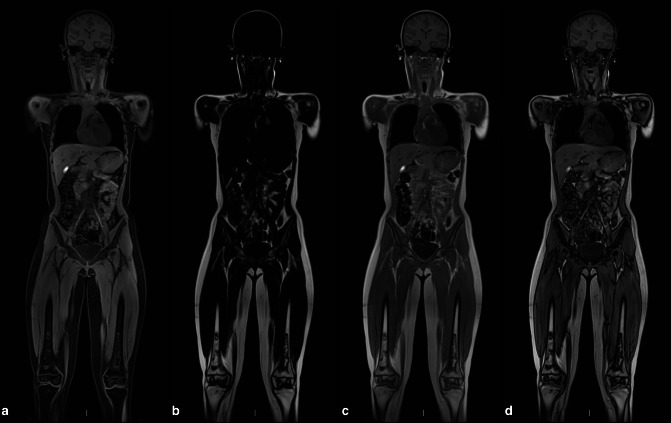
Whole-body coronal T1 Dixon MRI examinations showing water only (A), fat only (B), in-phase (C) and out-of-phase (D) sequences. Focal regions of medullary osteonecrosis can be seen in the distal femoral metaphases and epiphyses. The bone marrow otherwise returns normal signal with homogeneous high T1 signal in the fat-only, in-phase and out-of-phase sequences.
